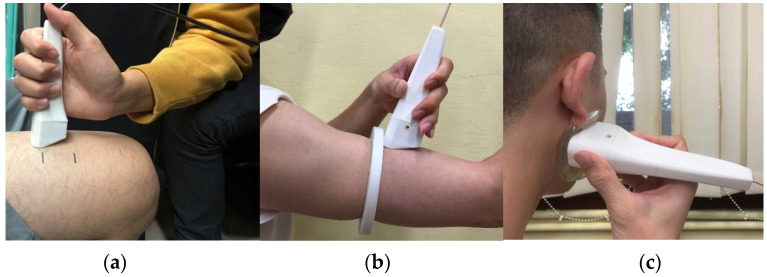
Ultrasound scanner measurement on subjects (a) thigh, (b) upper arm, and (c) cheek.
